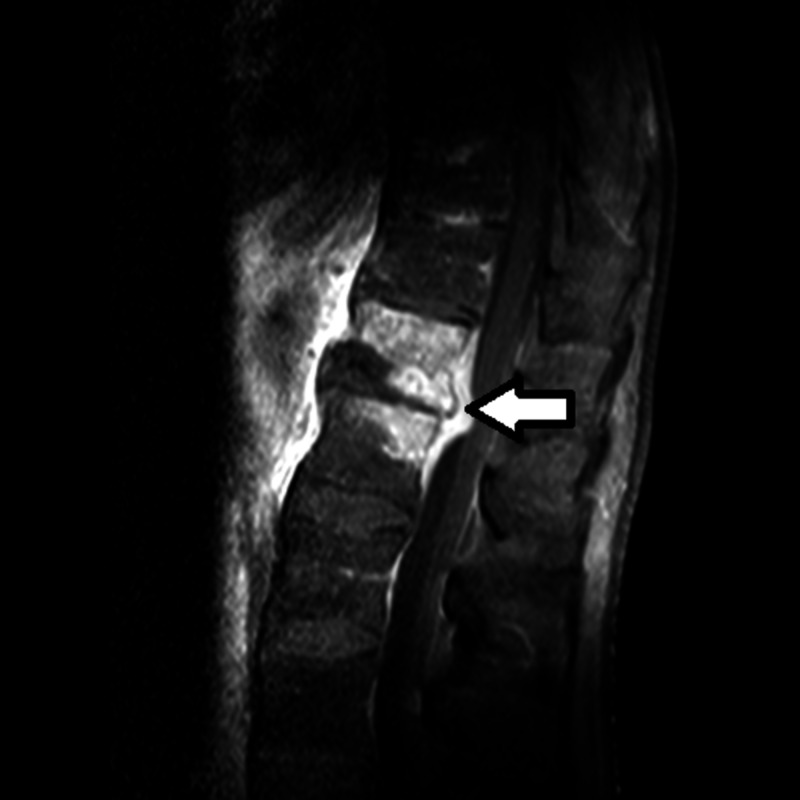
MRI of thoracolumbar area. Image shows abnormal enhancement at level T12-L1 consistent with discitis and osteomyelitis.
